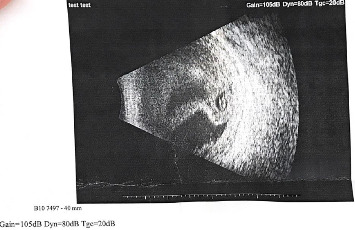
Ultrasound of the left eye: This image shows inflammatory debris present—the vitreous chamber shows dense vitreous opacities with signs of retinal detachment. Inflammatory debris likely indicating vitritis, a key feature of endophthalmitis.
